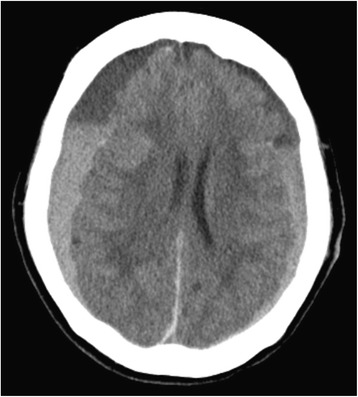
Brain computed tomography on the operative day. A large right subdural hematoma with secondary severe midline shift was observed
